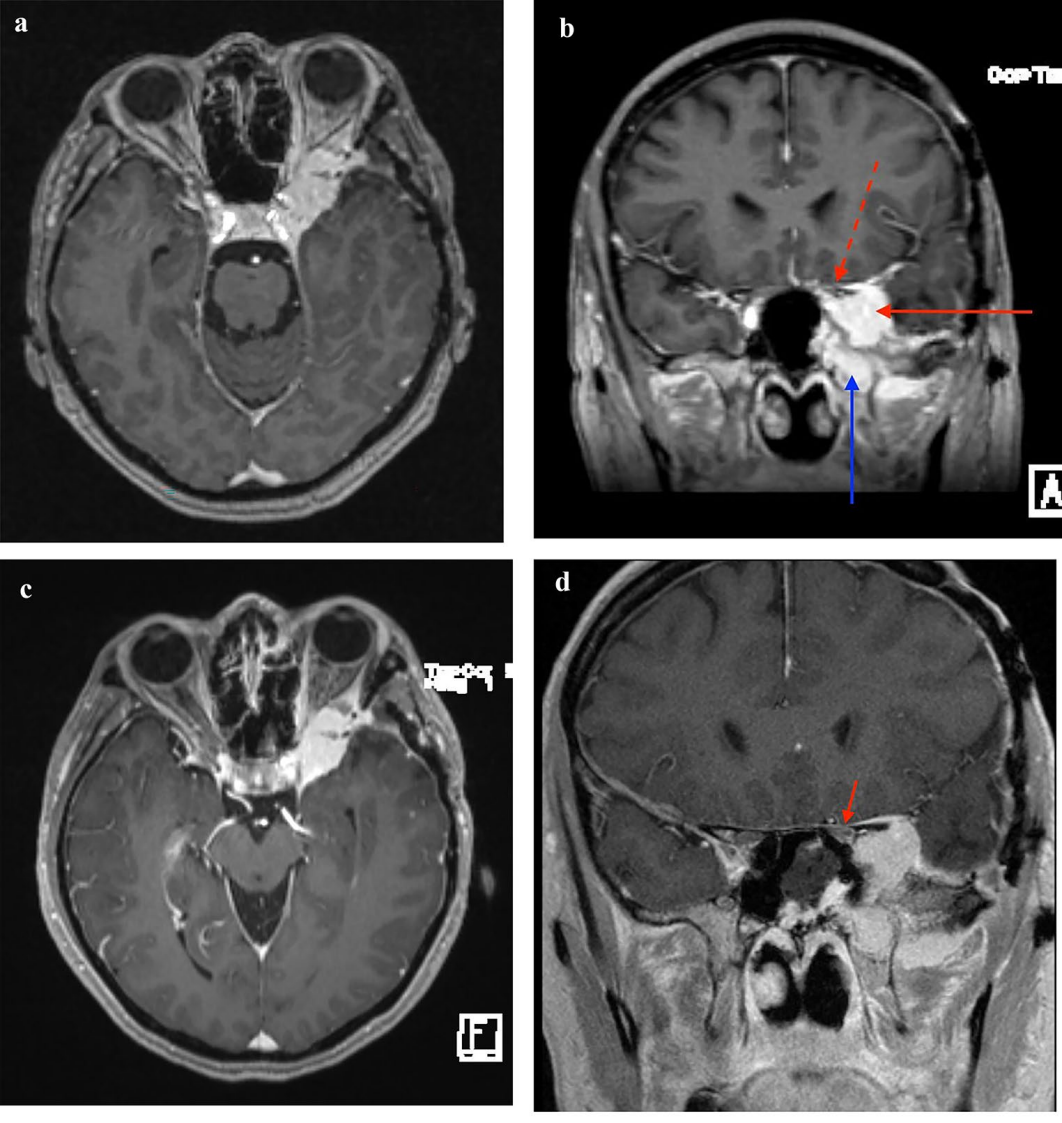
Brain magnetic resonance imaging of the patient. (A) Pre-operative axial image demonstrates significant orbital invasion via the superior orbital fissure (B) Pre-operative coronal image demonstrating the significant lateral cavernous sinus component of the tumor (solid red arrow), tumor bulk within the pterygopalatine fossa/infraorbital space (solid blue arrow), as well as optic nerve compression (dashed red arrow) (C) Post-operative axial image demonstrating no significant debulking of the intraorbital tumor (D) Post-operative coronal image 180 degree decompression of the left optic nerve, medial to the tumor bulk (solid red arrow)
